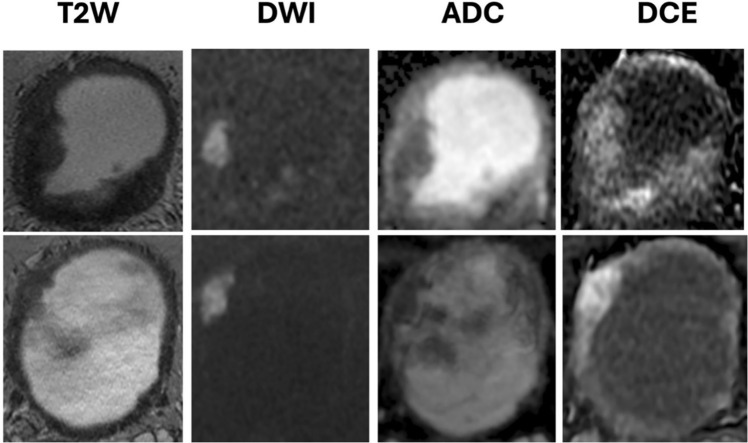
Case example of a VI-RADS 4 in a Sarcopenic 67 years old male patient (SMI value = 41.43 cm^2^/m^2^) with a sessile lesion located on the right lateral bladder wall. After neoadjuvant chemotherapy, the lesion still infiltrates the muscularis propria layer. Showing no downstaging and no measurable reduction in size. Therefore, it indicates a NacVI-RADS score of 5. T2W: T2-weighted imaging; DWI: diffusion-weighted imaging; ADC: apparent diffusion coefficient); DCE: dynamic contrast enhancement)
